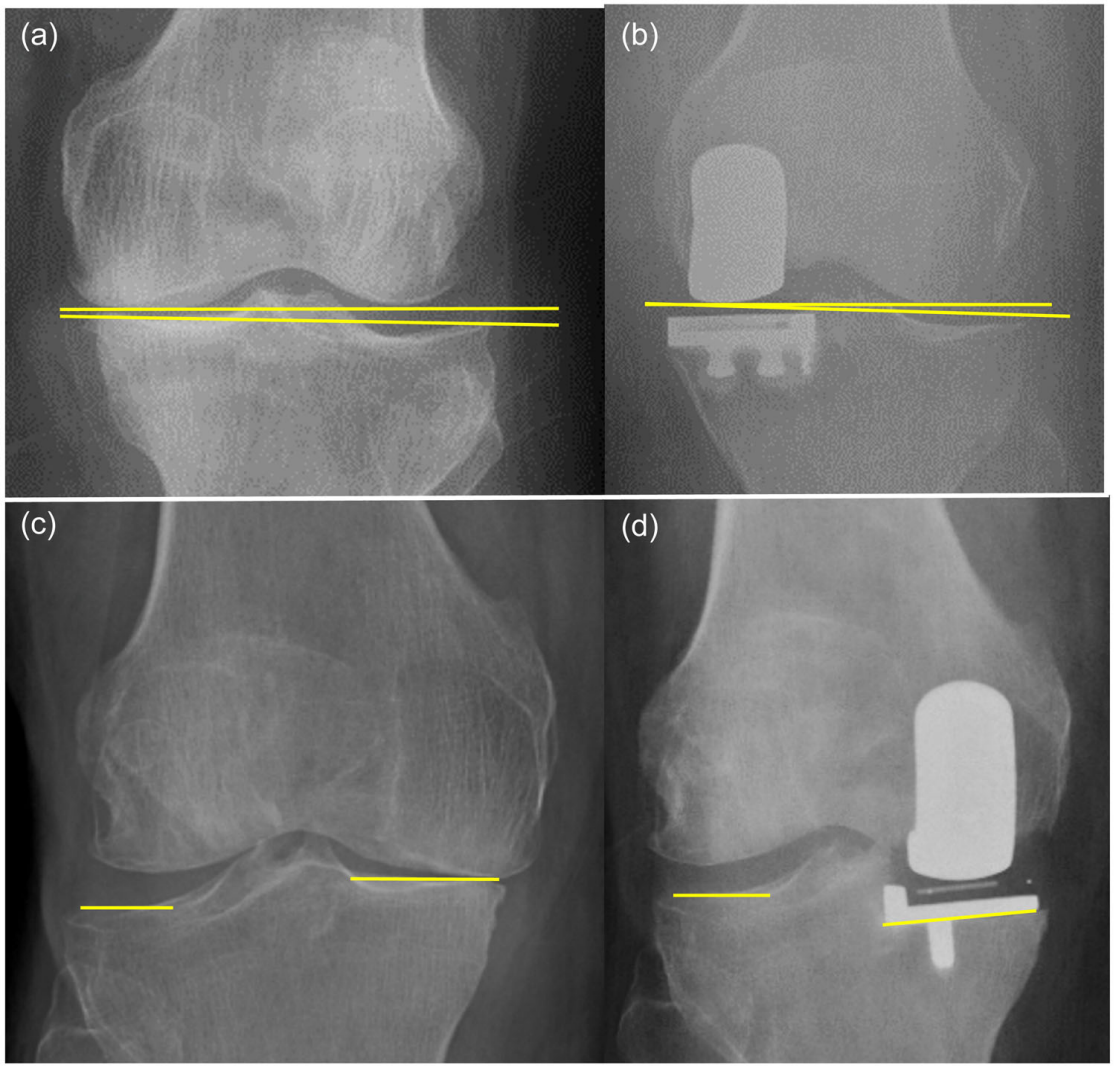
Measurement of joint line obliquity angle (a and b) and angle between the medial and lateral joint lines (c and d) on antero‐posterior direct knee radiographs.
